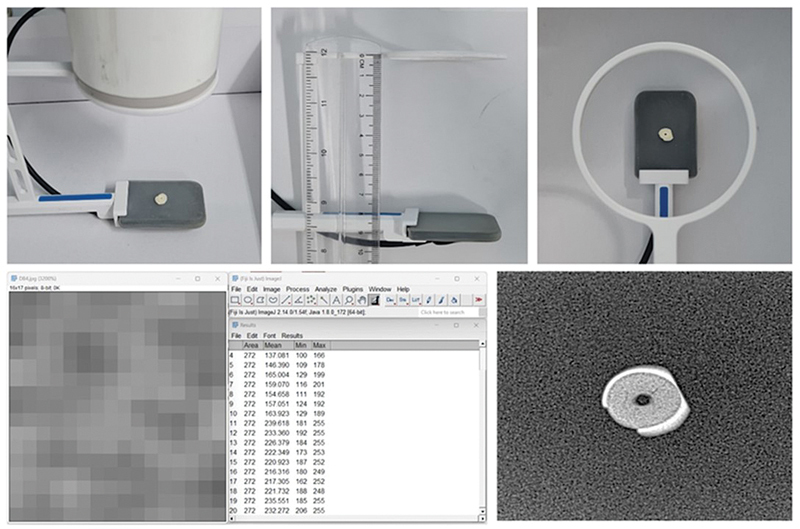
Setup process for radiographic imaging of tooth slices and analysis of gray values using Image J.
